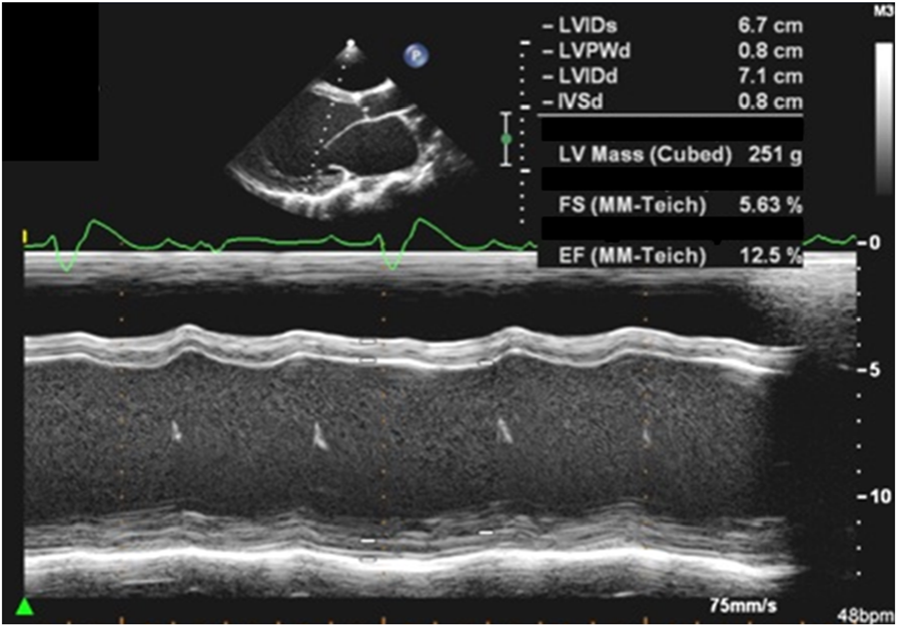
M-mode echocardiographic recordings from the index patient showing severe dilatation of the left ventricle (LVIDs: Left ventricle internal diameter during systole; LVIDd: Left ventricle internal diameter in diastole; both are markedly increased) and poor contractility with an ejection fraction (EF) of 12.5 %
